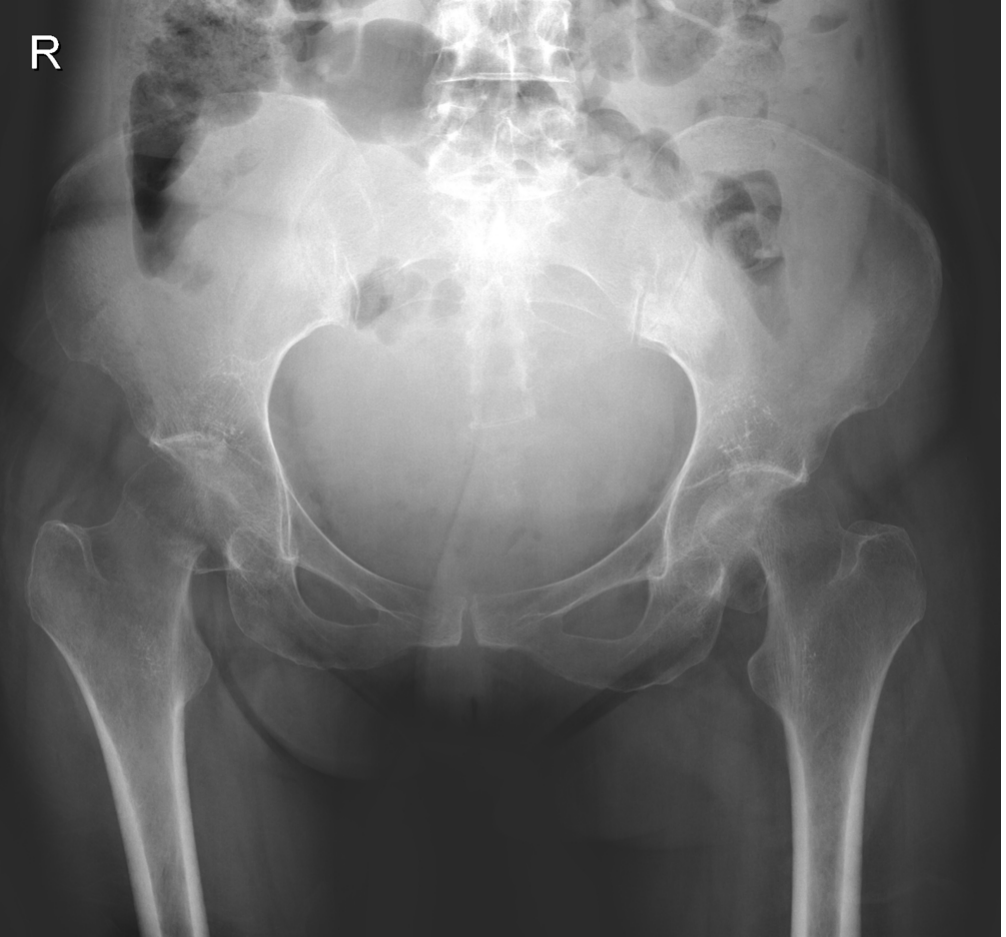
Antero-posterior radiograph of the pelvis post partum.
